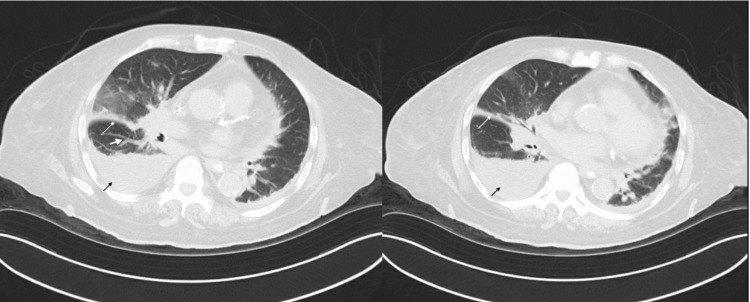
Computed tomography of the chest, abdomen, and pelvis without contrast Worsening right-side pleural effusion (black arrow) and pleural thickening along the major fissure (thin white arrow) with irregular reticular markings (thick white arrow).
